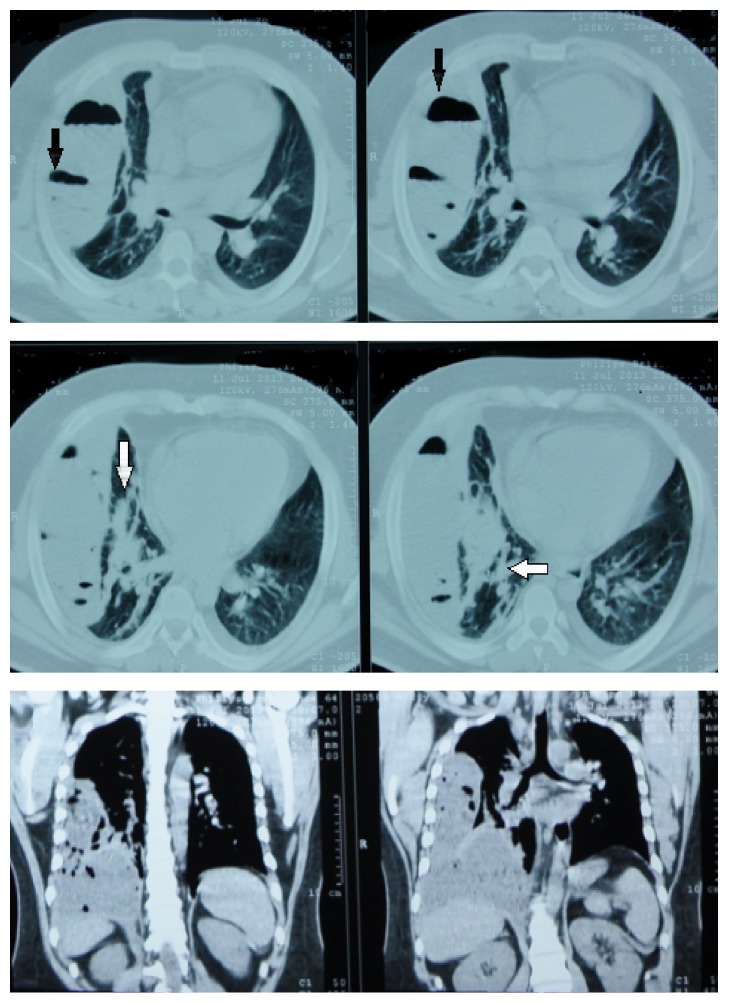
Chest CT scan right sided pleural effusion with pocket mainly in lateral aspect and in the oblique fissure, multiple gas bubbles with air fluid levels (black arrow), and partial atelectasis of right middle and lower lobes that are medially displaced (white arrow).
